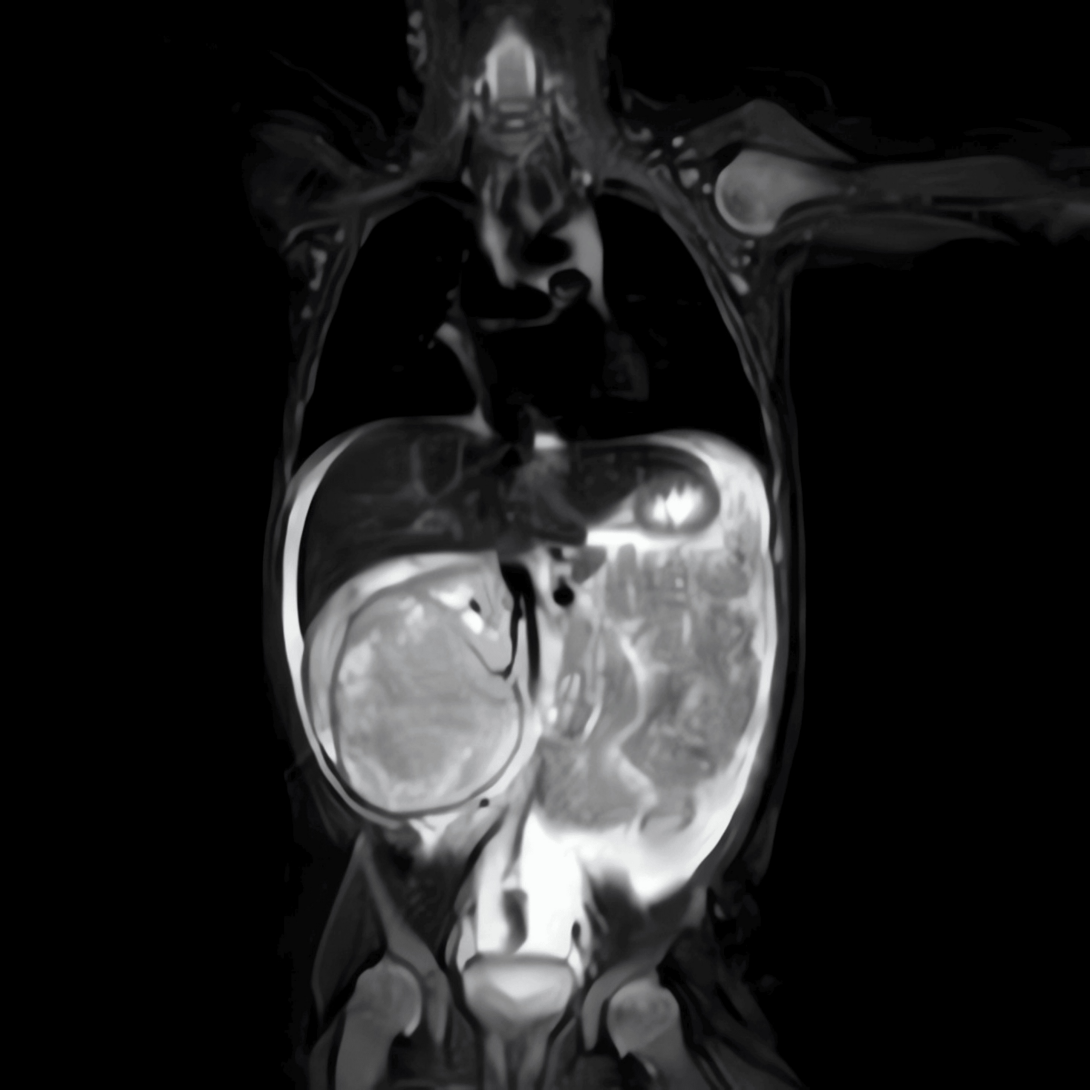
Coronal MRI of the abdomen demonstrating the large right renal tumor displacing surrounding structures
